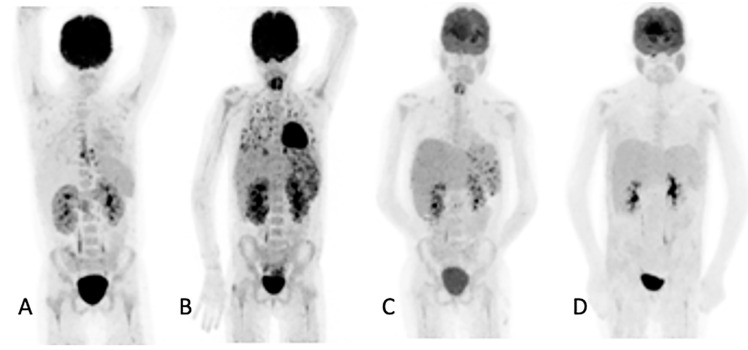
Ten-year-old female with acute myeloid lymphoma who developed a fever, on chemotherapy, with no response to antibiotics. An abdominal ultrasound demonstrated bilaterally enlarged kidneys. Initial [^18^F]FDG PET/CT MIP image (A) showed focal metabolic uptake in kidneys, spleen and esophagus. She was started on antifungal treatment, but the fever persisted, and a repeat study (B) showed the worsening of renal splenic lesions with no pulmonary lesions. The antifungal treatment was changed; the fever subsided, and the serum infective markers decreased. A repeat [^18^F]FDG PET/CT performed after 6 weeks (C) showed resolution of the pulmonary and most of the renal and splenic lesions. The treatment was continued for another month, and a follow-up [^18^F]FDG PET/CT (D) showed a complete metabolic response that helped the clinician to end the antifungal treatment.
